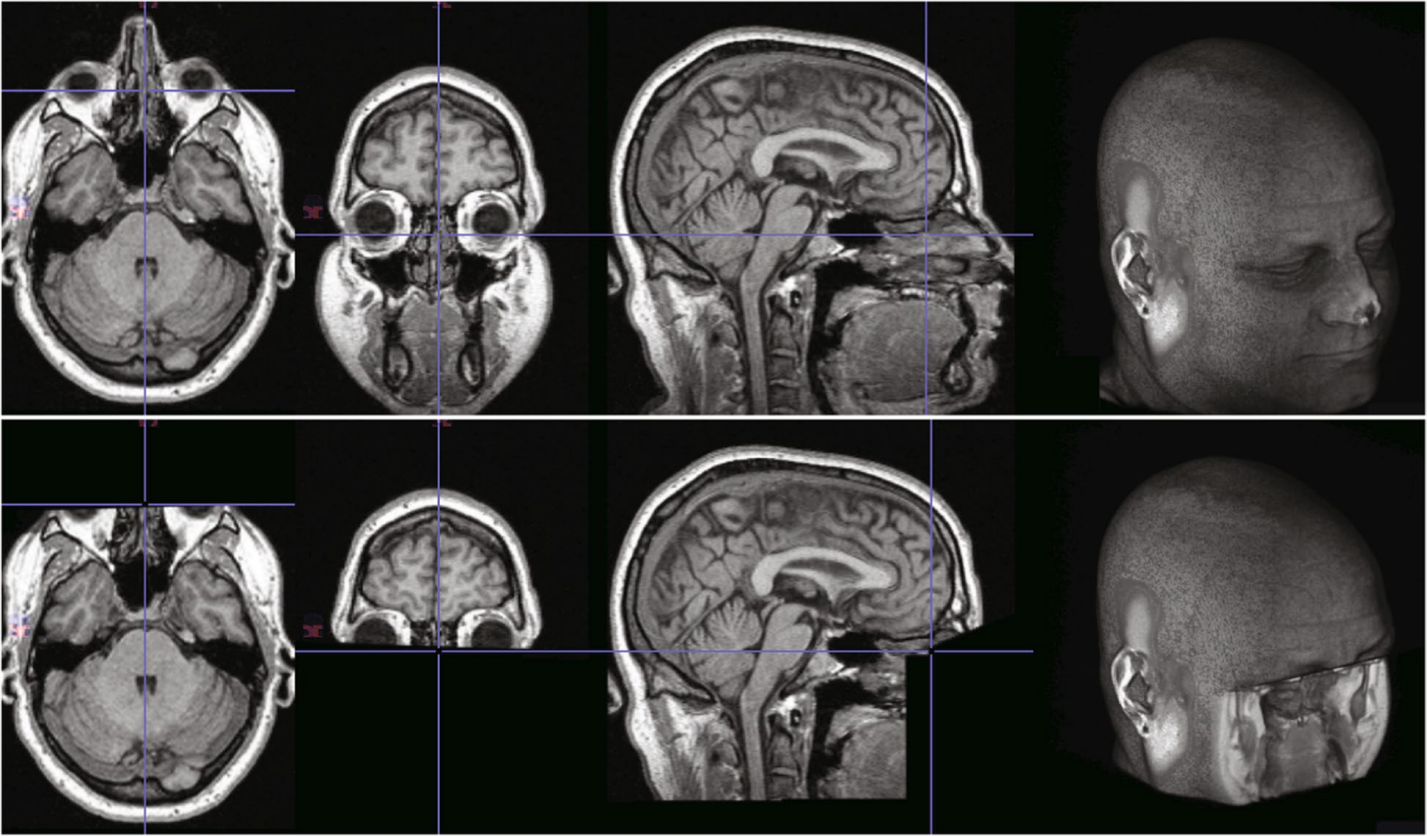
Example of face removal from MRI scans of the head obtained with “Pydefacer”. The first row depicts the original images, while the second shows the same images after the removal of the face. Reprinted from [22] (no permission required)
